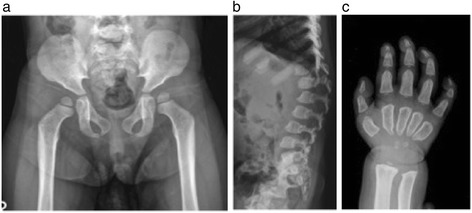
Radiographs of a two-year-old female patient diagnosed with Hurler syndrome (MPS IH) (a-c).
